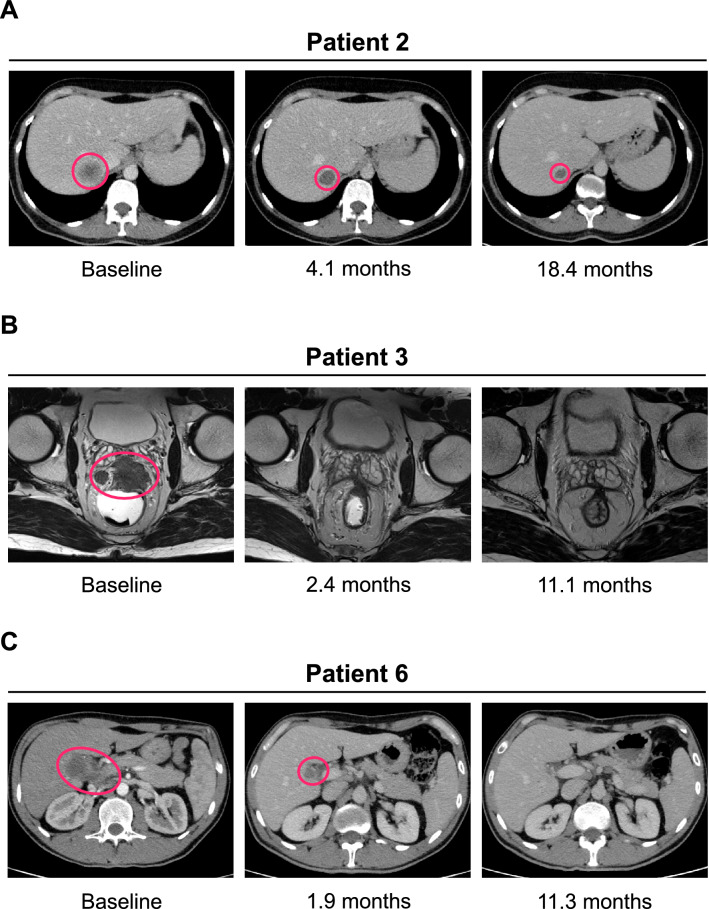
Radiographic response of the liver metastasis of patient 2 (a), pelvic metastasis of patient 3 (b), and liver metastasis of patient 6 (c). CT scans at baseline prior to PD-1 inhibition and follow-up CT scans are shown for each patient. Circled areas indicate the respective tumor lesions
